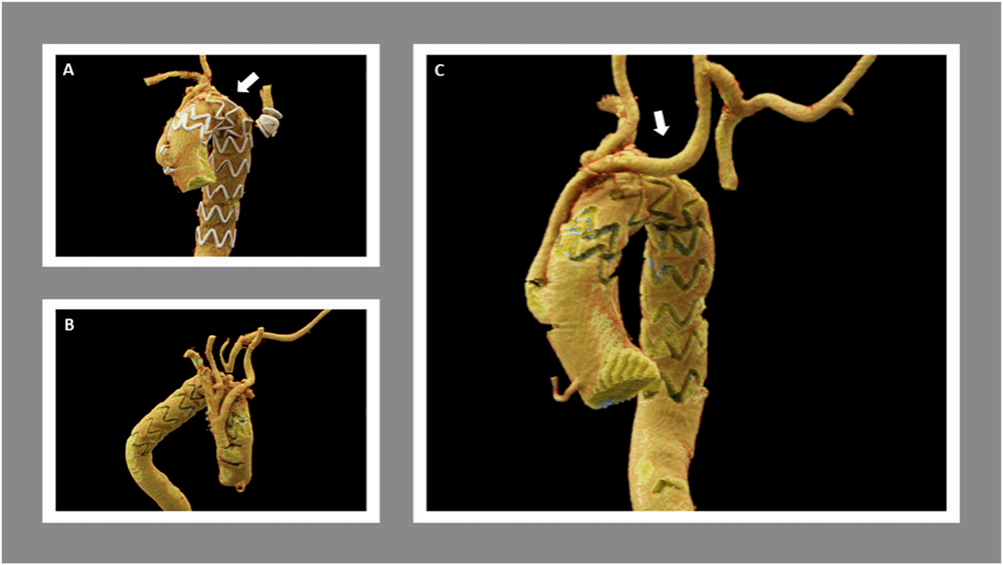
Six months follow-up computed tomography angiogram findings. Left anterolateral view (A), right lateral view (B), and left anterolateral view (C) of the three-dimensional cinematic volume rendering technique demonstrates right-sided aortic arch status post endovascular repair with reimplantation of common trunk supplying the innominate and left common carotid arteries (widely patent). The left common carotid to left axillary bypass graft is widely patent and exclusion the left subclavian artery at the origin with Amplatz plug. The artery distal to this is widely patent. The remaining portions of the visualized great vessels are widely patent. Type II endoleak arising from the first right posterior intercostal artery (arrows).
